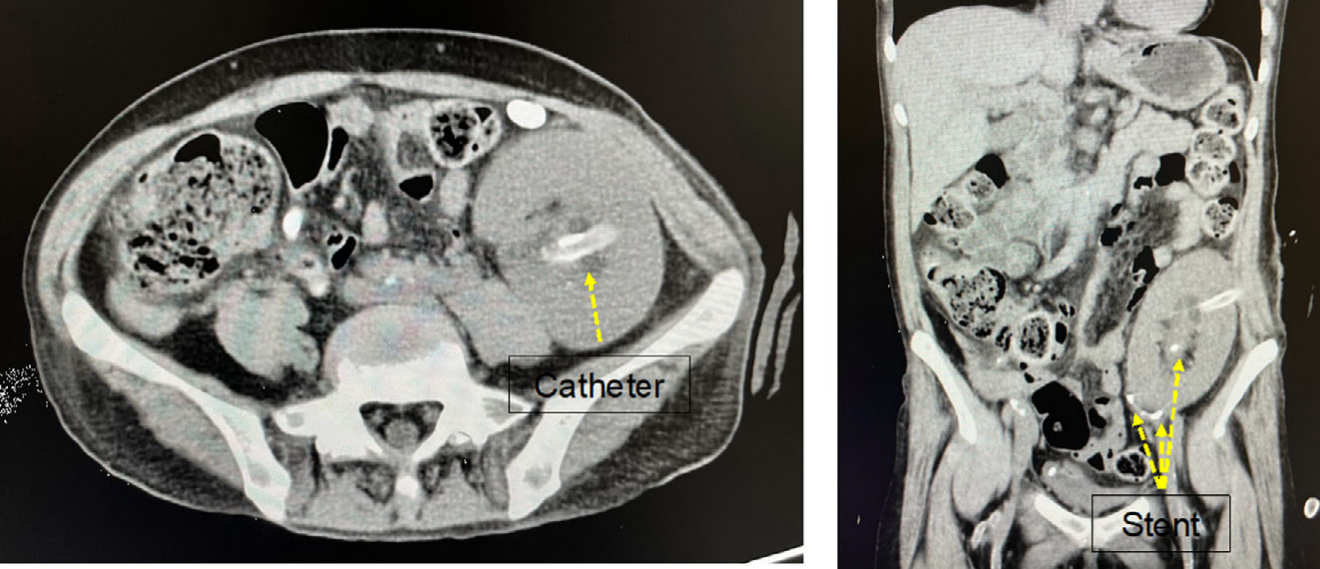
Postoperative CT images. On postoperative Day 1, CT shows that both the nephrostomy catheter and ureteral stent were in place. No residual fragments were observed. The nephrostomy catheter was subsequently removed.
